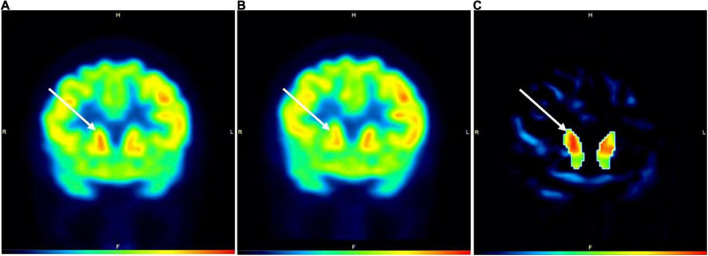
PET images during active (A) and SHAM (B) conditions in Subject3. Image (C) represents a subtraction PET image with the globally normalized activity in sham subtracted from the globally normalized activity in active. All areas in image (C) are masked except for the caudate nuclei. White arrows denote the location of the caudate nuclei. The color bar indicates level of increased FDG uptake (black = no uptake; red = highest glucose uptake). H = head; F = foot; R = right; L = left.
